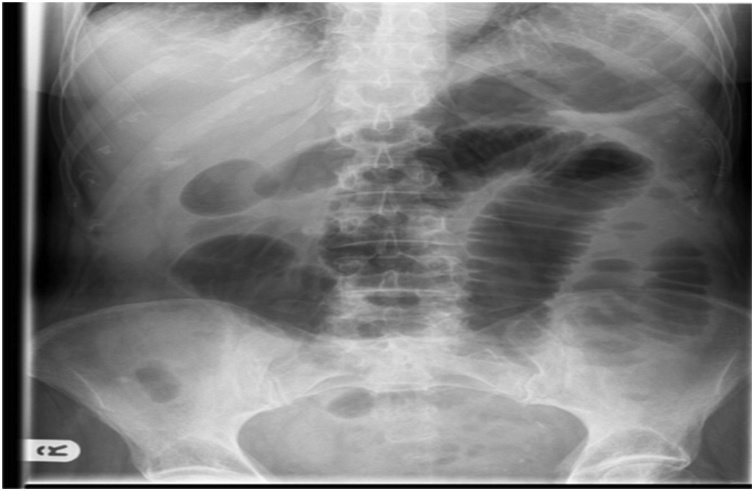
X-ray of abdomen show multiple dilated small gut loops in central part of abdomen.
